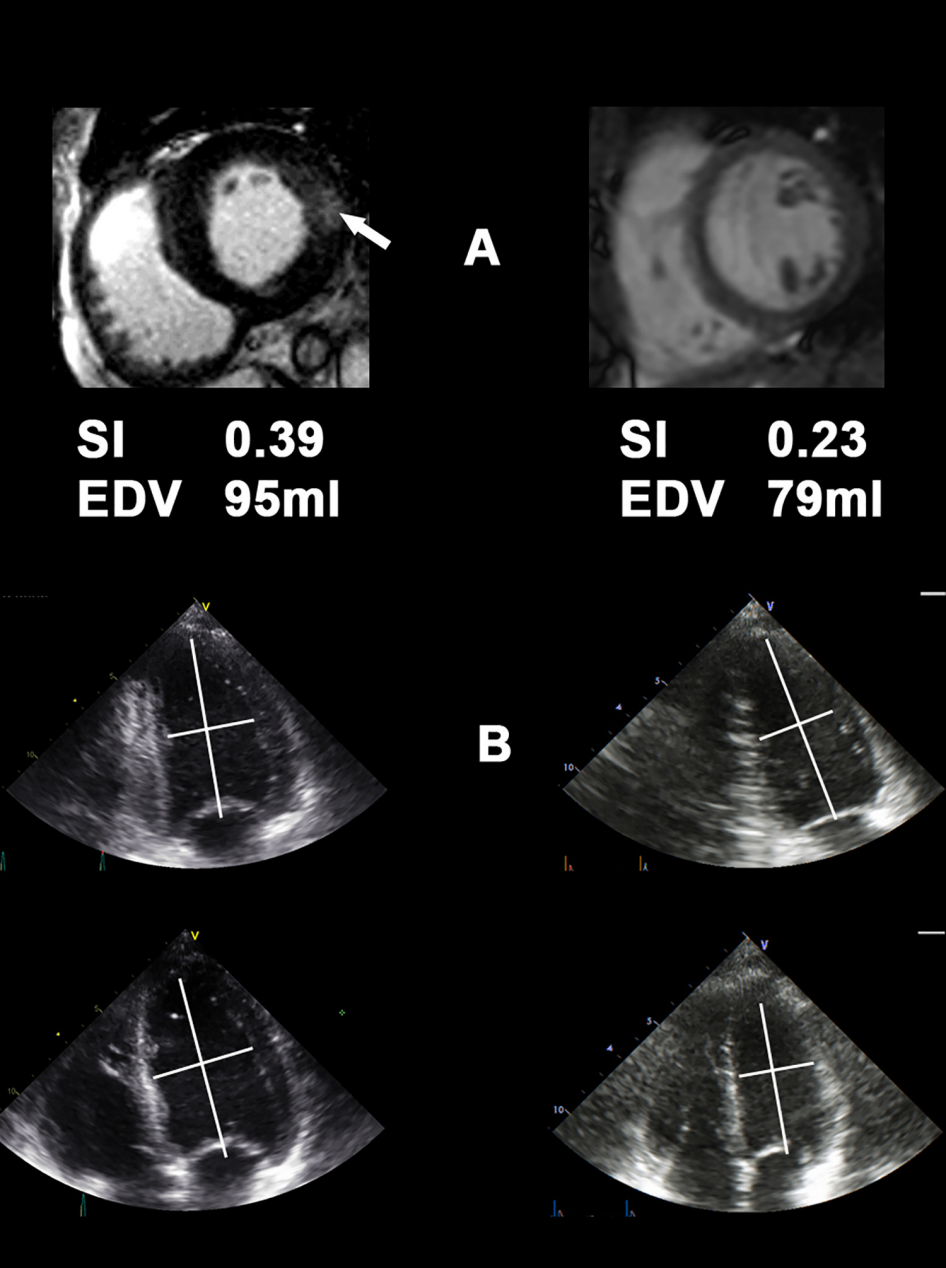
Typical cardiac magnetic resonance imaging (cMRI) (A) and echocardiography (B) of patients with normal sphericity index (SI; right row) and elevated SI (left row). Note the pathological late enhancement (LE; white arrow) in the cMRI and the more spherical shape of the LV in the left row. SI; sphericity index, EDV; end-diastolic volume.
